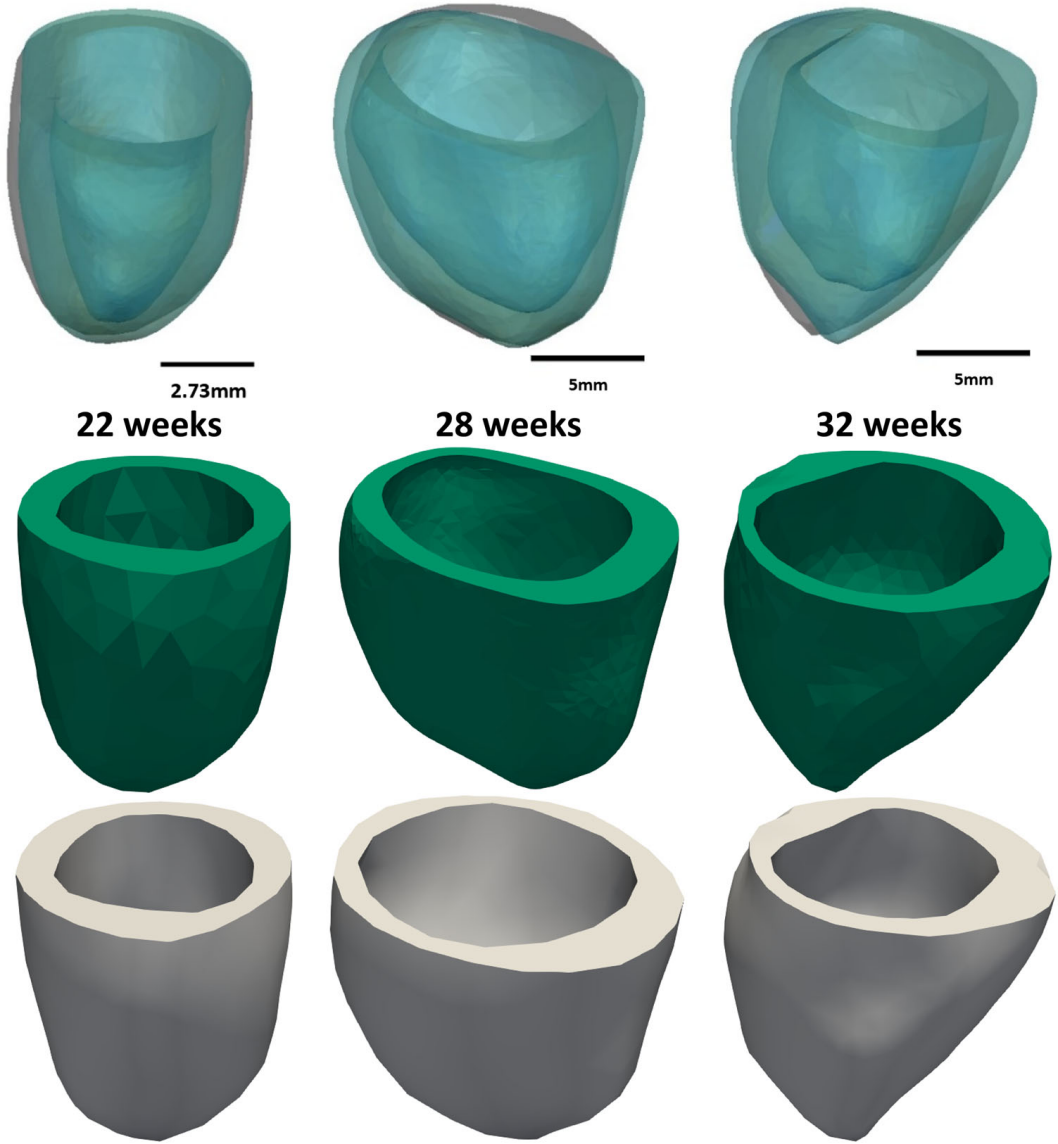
The end-systolic LV geometries of the healthy fetal hearts studied, obtained from FE modelling (green), and from ultrasound image motion tracking (gray). Geometries from both analyses showed a good match. The input to both FE modelling and motion tracking were the segmentation of the end-diastolic LV geometry.
